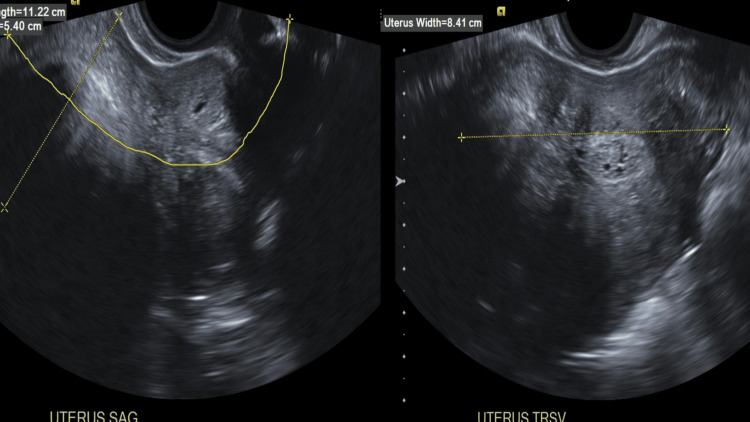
A transvaginal ultrasound (sagittal and transverse view) revealed an enlarged uterus measuring 11.2 x 5.4 x 8.4 cm with heterogeneous myometrial echotexture.
